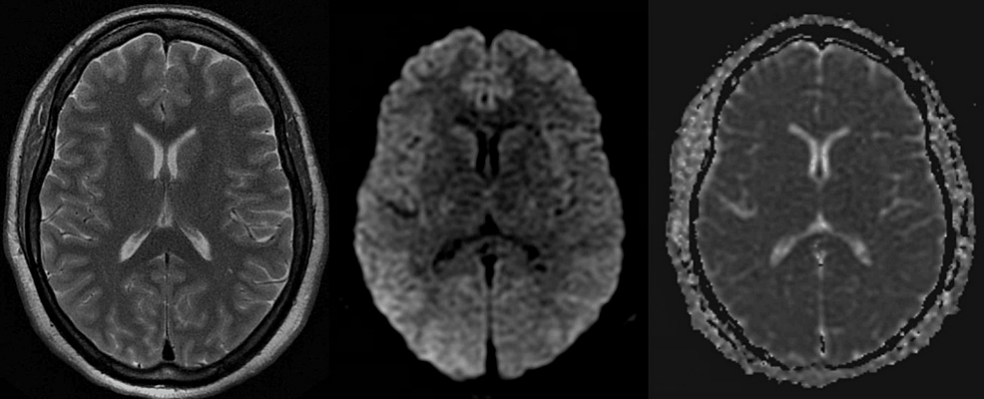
Follow-up MRI of the brain on day four The initial findings of diffuse cerebral edema and infarctions, as depicted in Figure 2, have resolved. Left: T2-weighted image; Middle: diffusion-weighted image; Right: apparent diffusion coefficient image
